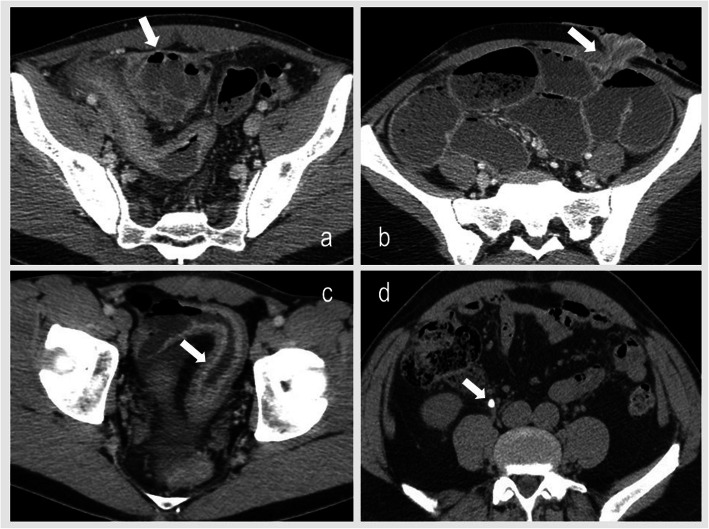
Examples of diagnosis made by CT scan: a abdominal abcess in a 36-years old patient (white arrow); b bowel obstruction related to a stenosis of the stomia in a 25-years old patient (white arrow); c ileal inflammation in a 21-years old patient (white arrow) and d right ureteral lithiasis in a 48-years old patient (renal colic)
